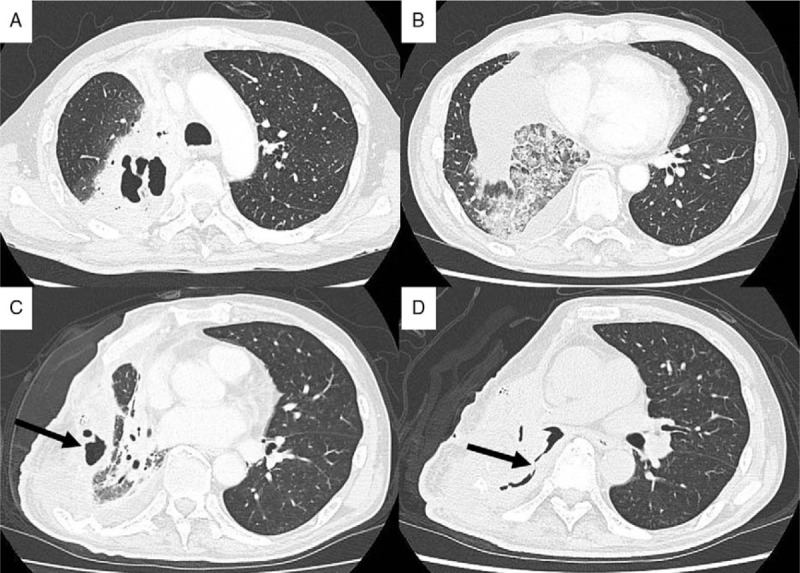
(A) Chest computed tomography (CT) revealed a cavitary lesion, suggesting a bronchopleural fistula with empyema. (B) Chest CT showed subsequent aspiration pneumonia in the right lower lobe. (C) Chest CT taken before the endobronchial procedure revealed the cavitary lesion (black arrow), suggesting a persistent bronchopleural fistula. (D) A CT image taken before the endobronchial procedure showed the fistula, in which the pleural cavity and bronchial tree communicated (black arrow).
